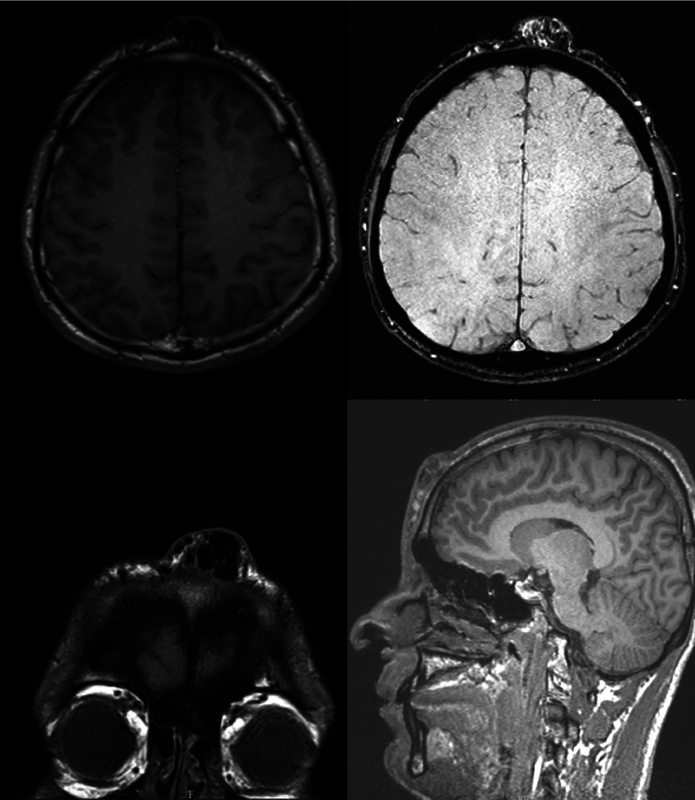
MRI reveals a high-flow extracranial arteriovenous malformation in the subcutaneous forehead tissues. The nidus is supplied by tortuous bilateral superficial temporal arteries, with a prominent midline draining vein coursing into the angular and facial veins. No intracranial extension or parenchymal abnormalities are seen. MRI, magnetic resonance imaging.
